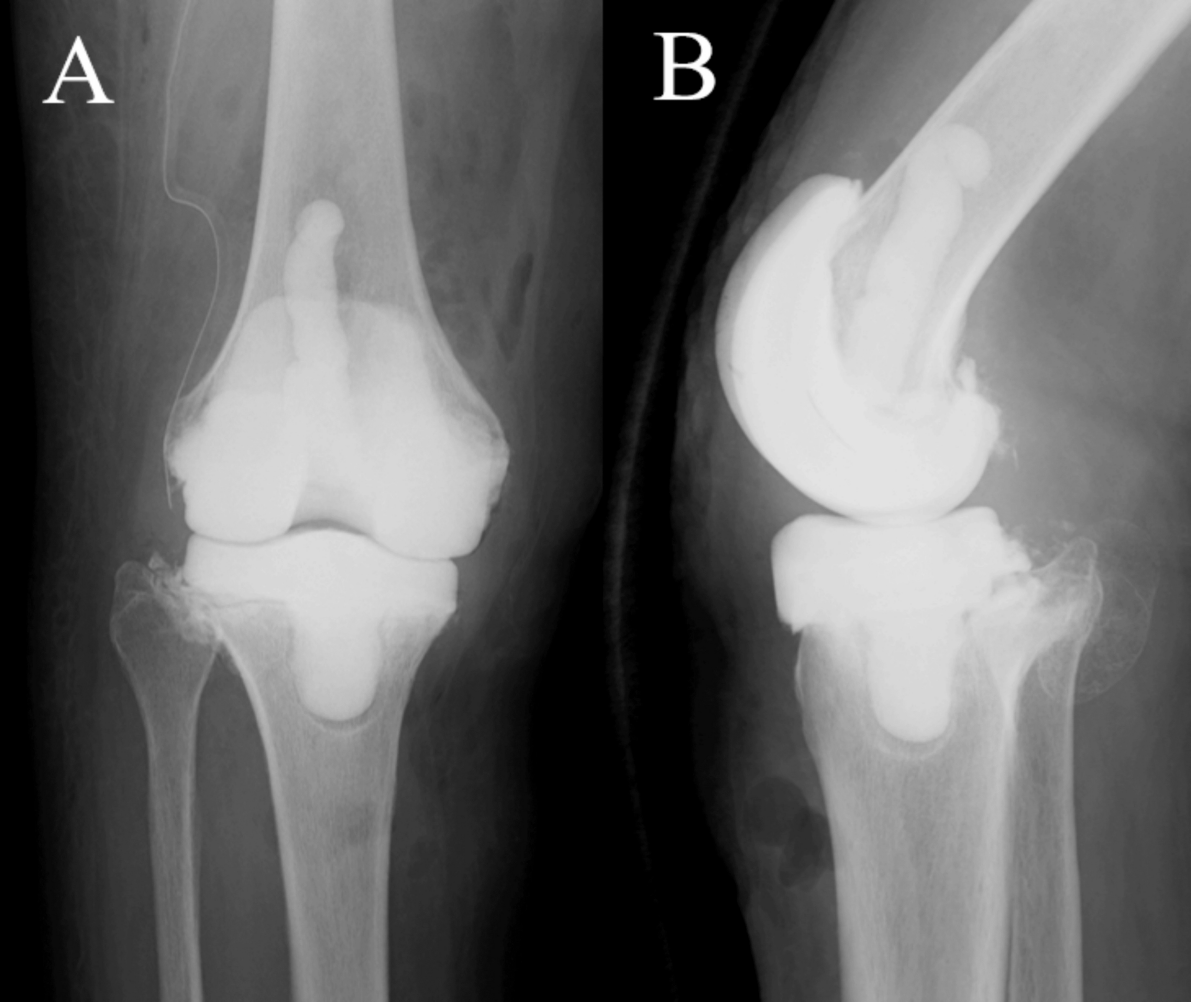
After antibiotic-loaded cement spacer replacement Uncontrolled infection led to removal of the implant and antibiotic-loaded cement spacer replacement, which was ineffective.  (A) Anteroposterior radiograph of the knee joint. (B) Lateral radiograph of the knee joint.
